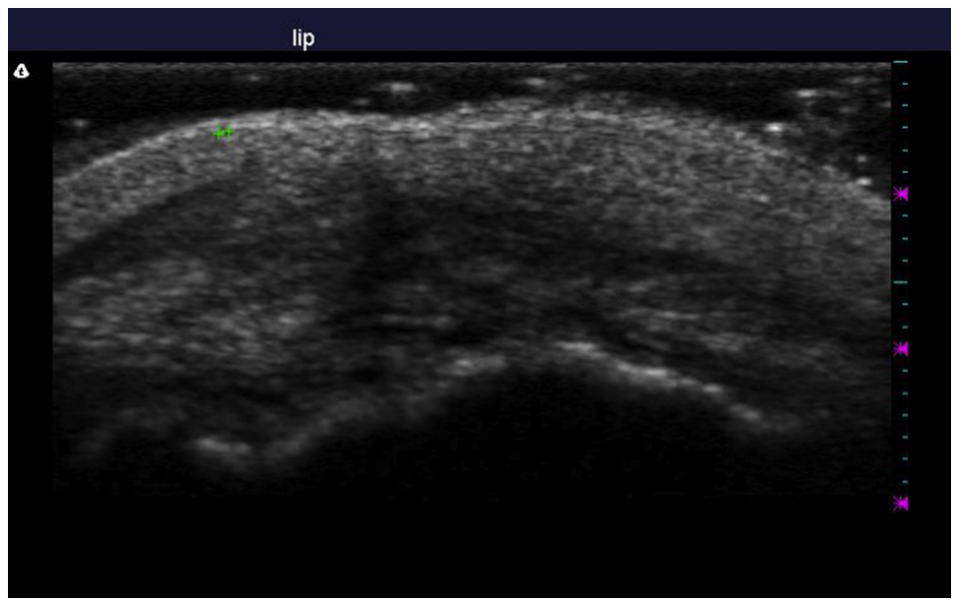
The width of upper lip scar was measured by ultrasound, as indicated by the two green crosses.
